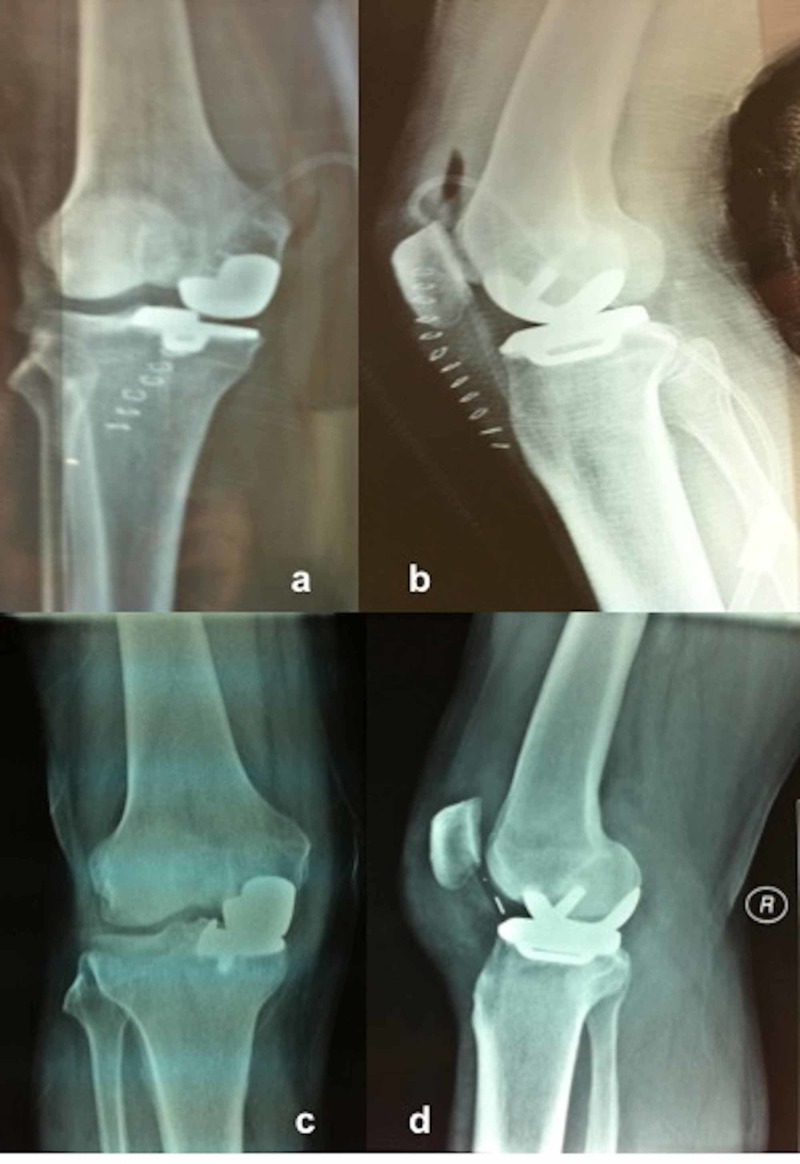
A 60-year-old male patient with UKA due to medial compartment osteoarthritis a,b) Postoperative radiographs of the right knee; c,d) Radiographs of mobile-bearing dislocation at six weeks postoperatively (radiopaque wire of the bearing is seen at the anterior aspect of the knee joint) UKA: unicompartmental knee arthroplasty
